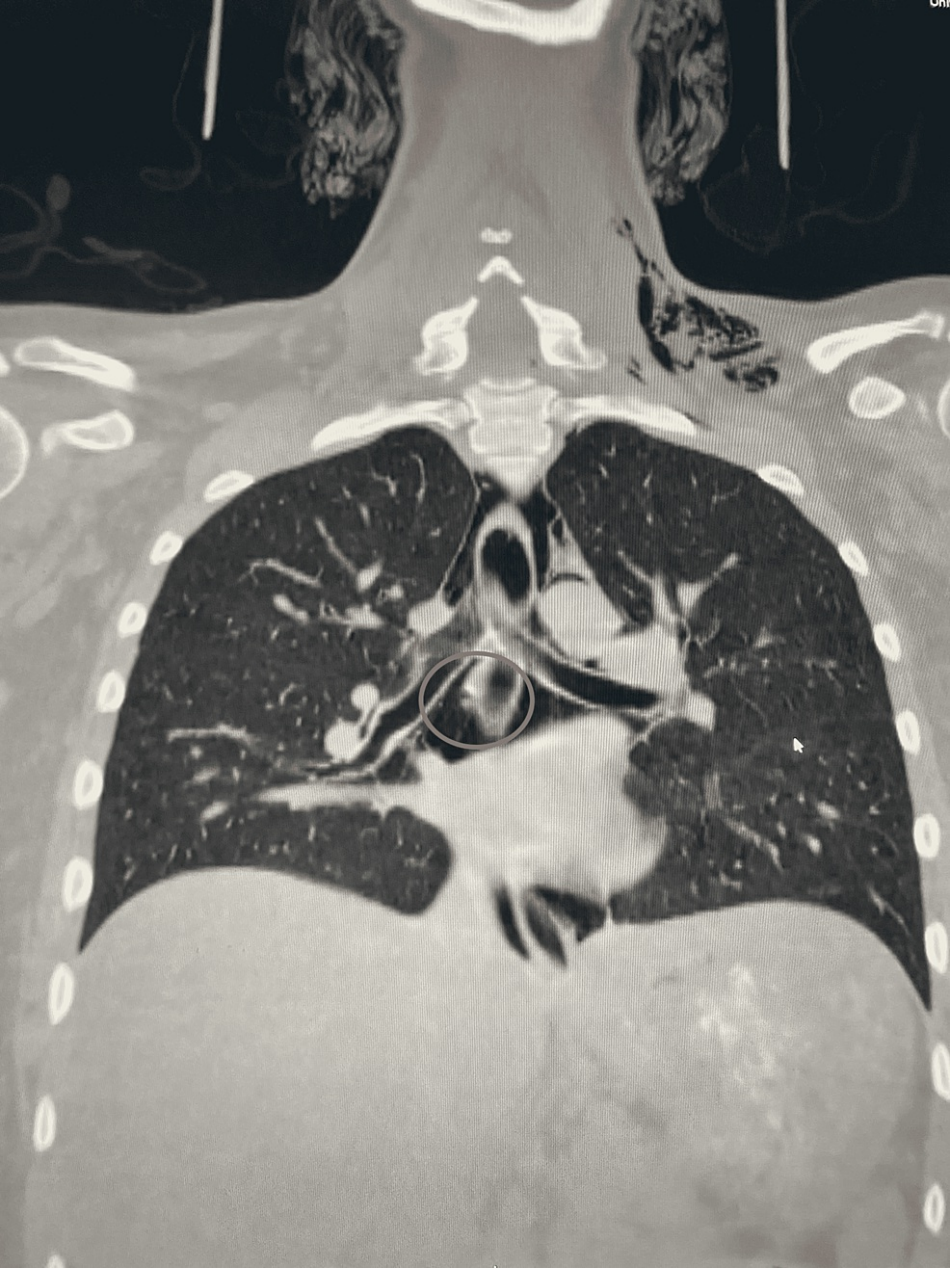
CT scan of head and neck The image shows a 2-mm tear of the right mid esophagus with extensive pneumomediastinum and subcutaneous gas at the base of the neck CT: computed tomography
